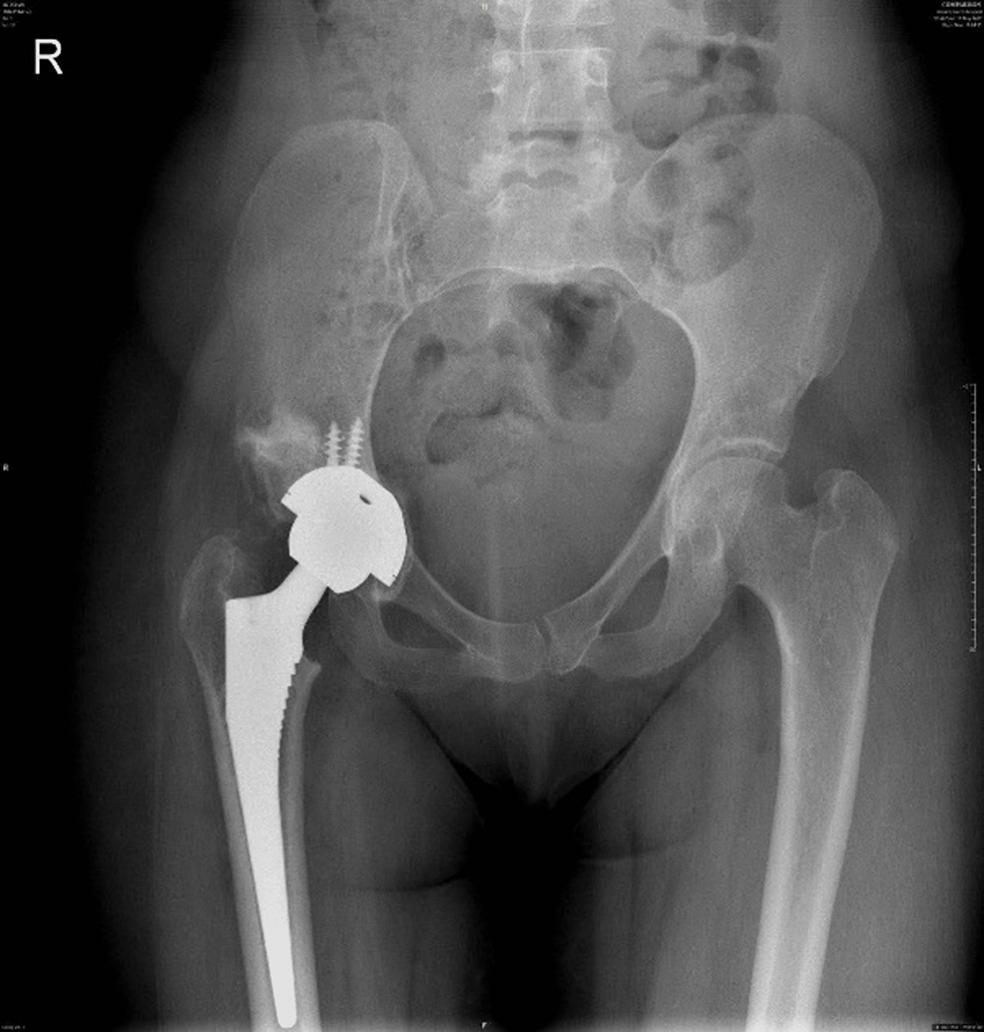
AP X-ray of the hip post operatively. AP: Anteroposterior.
